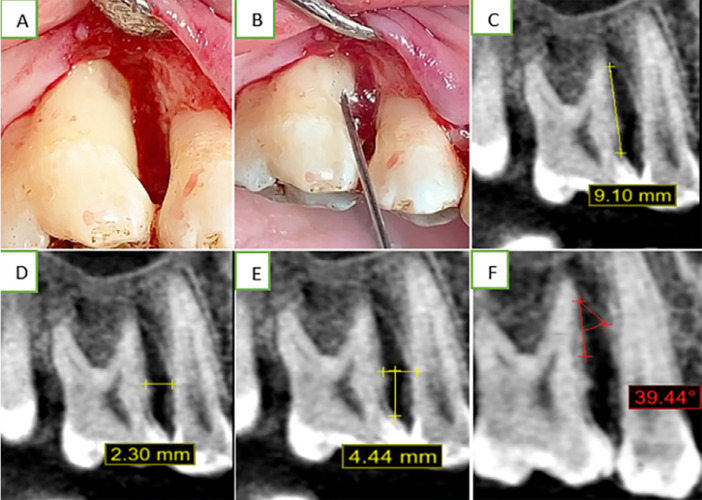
(A) MIST for 2-wall intrabony defect incision; (B) RGD loaded hydrogel application; (C) baseline radiographic measures of CEJ-BD= 9.10 mm; (D) radiographic width of bone defect= 2.3 mm; (E) radiographic measures of CEJ-BC= 4.44 mm; (F) defect angle= 39.44°
